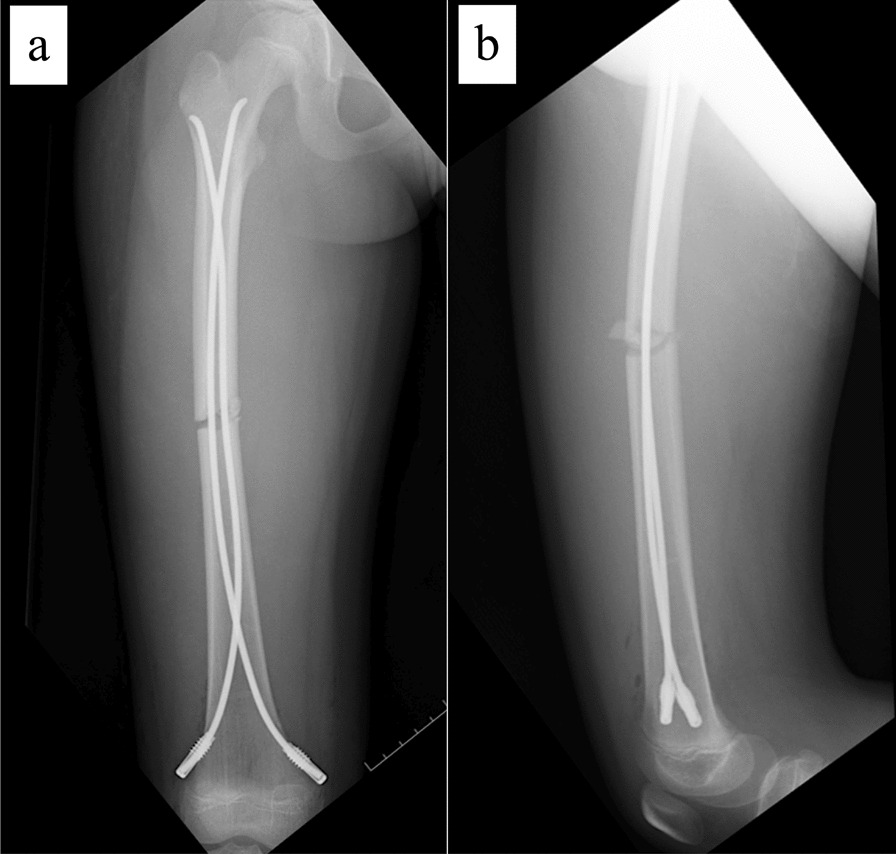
Postoperative radiographs of first surgery. a Anteroposterior image and b lateral image. Osteosynthesis was performed using two 3.5-mm-diameter nails inserted retrograde
